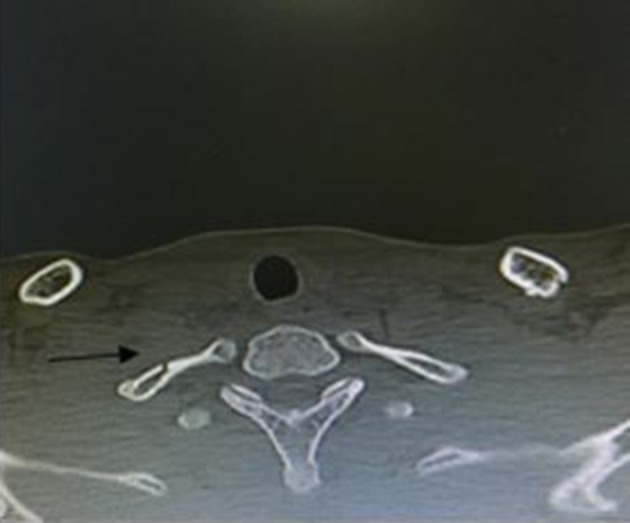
CT axial cut shows fracture in right first rib.
